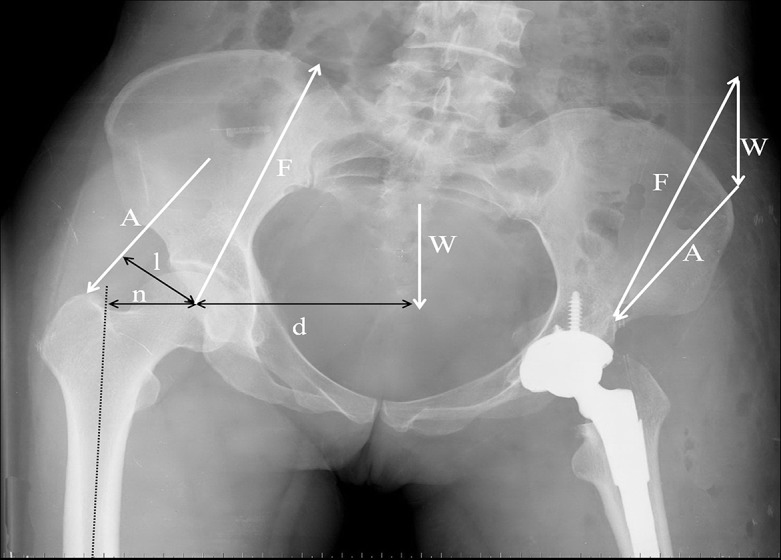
Radiograph showing the forces acting on the hip joint during a single-leg stance under equilibrium conditions. Gravitational force W, hip joint reaction force F, abductor force A, femoral shaft axial line S, abductor muscle moment arm l, force of gravity moment arm d, and femoral offset n.
